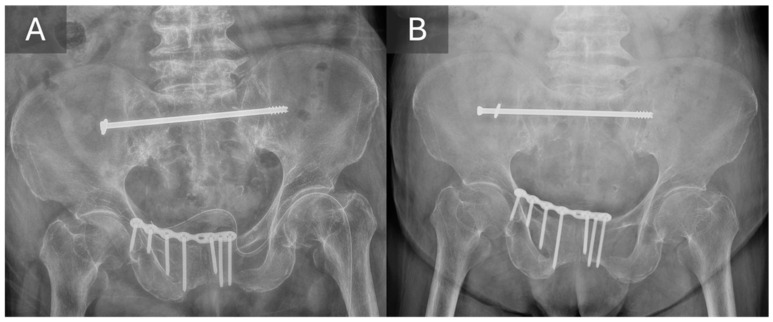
Immediate postoperative radiograph (A) and 6-month follow-up radiograph (B) from the same patient, demonstrating simple backout of TITS screw without secondary displacement. The alignment of the posterior pelvic ring was maintained.
